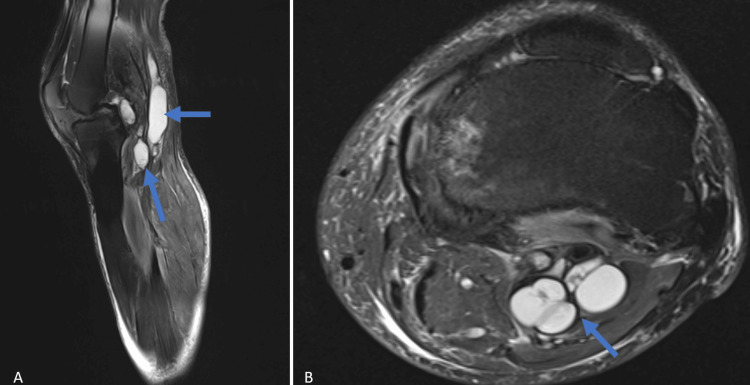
Preoperative fat-saturated T2-weighted MRI of the left knee A: Preoperative fat-saturated T2-weighted MRI of the left knee, sagittal scan, showing multiple hyperintense cystic lesions in the popliteal fossa (blue arrows). B: Preoperative fat-saturated T2-weighted MRI of the left knee, axial scan, showing two well-circumscribed, multilobulated hyperintense cystic lesions (blue arrow).
